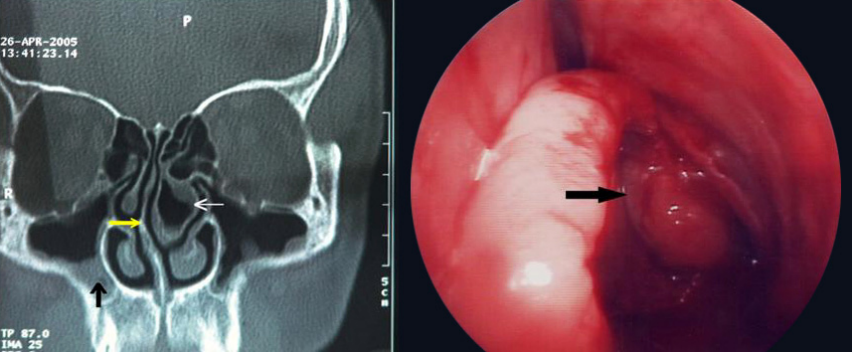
Left: Coronal computed tomography image shows right septal deviation (yellow arrow), left concha bullosa (thick white arrow), thickening of the mucosa covering the concha bullosa, and bilateral maxillary sinusitis (vertical black arrow). Right: Intraoperative views of the polyp in the left concha bullosa. (black arrow shows polyp)
